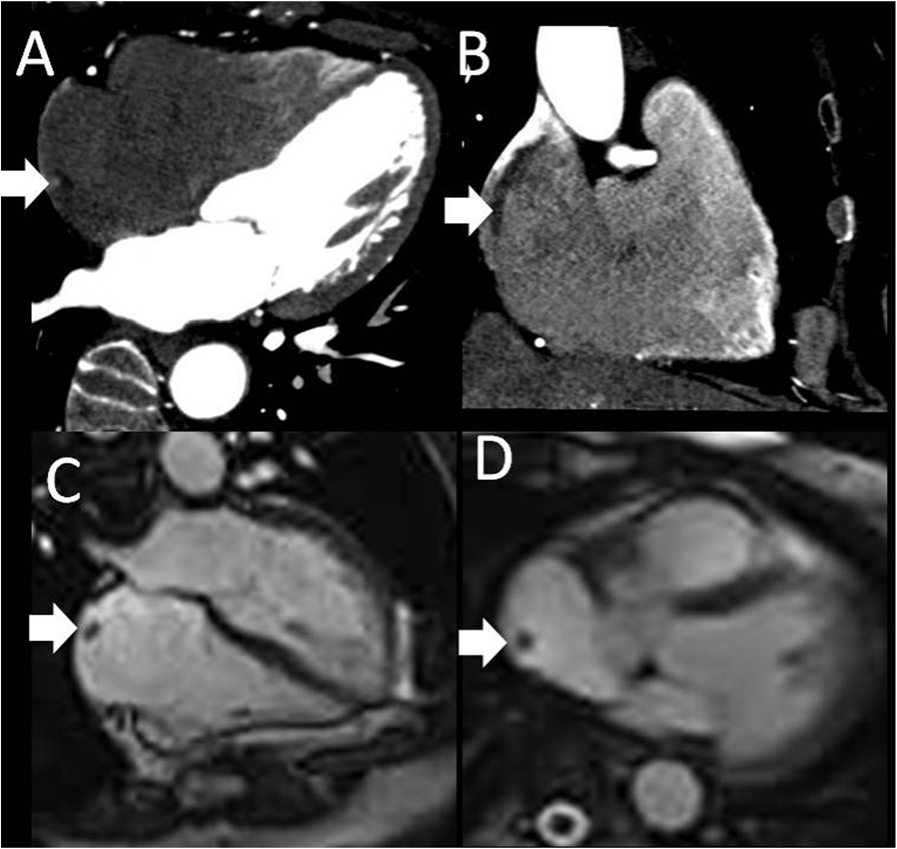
Computed tomography (A, B) and magnetic resonance (C, D) images of end-diastolic phase showing the prominent crista terminalis (arrows). In A and B the computed tomography images show the finding in four-chamber view and two-chamber long axis view, respectively. In C and D the steady-state free precession magnetic resonance images show the finding in four-chamber view and axial view, respectively.
